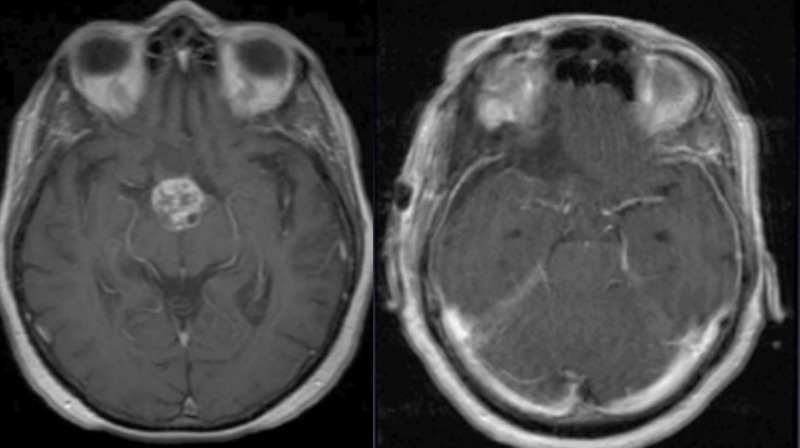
Comparison of preoperative and postoperative imaging The preoperative MRI is seen on the left, with the postoperative MRI on the right. The imaging comparison shows near complete total gross resection.
